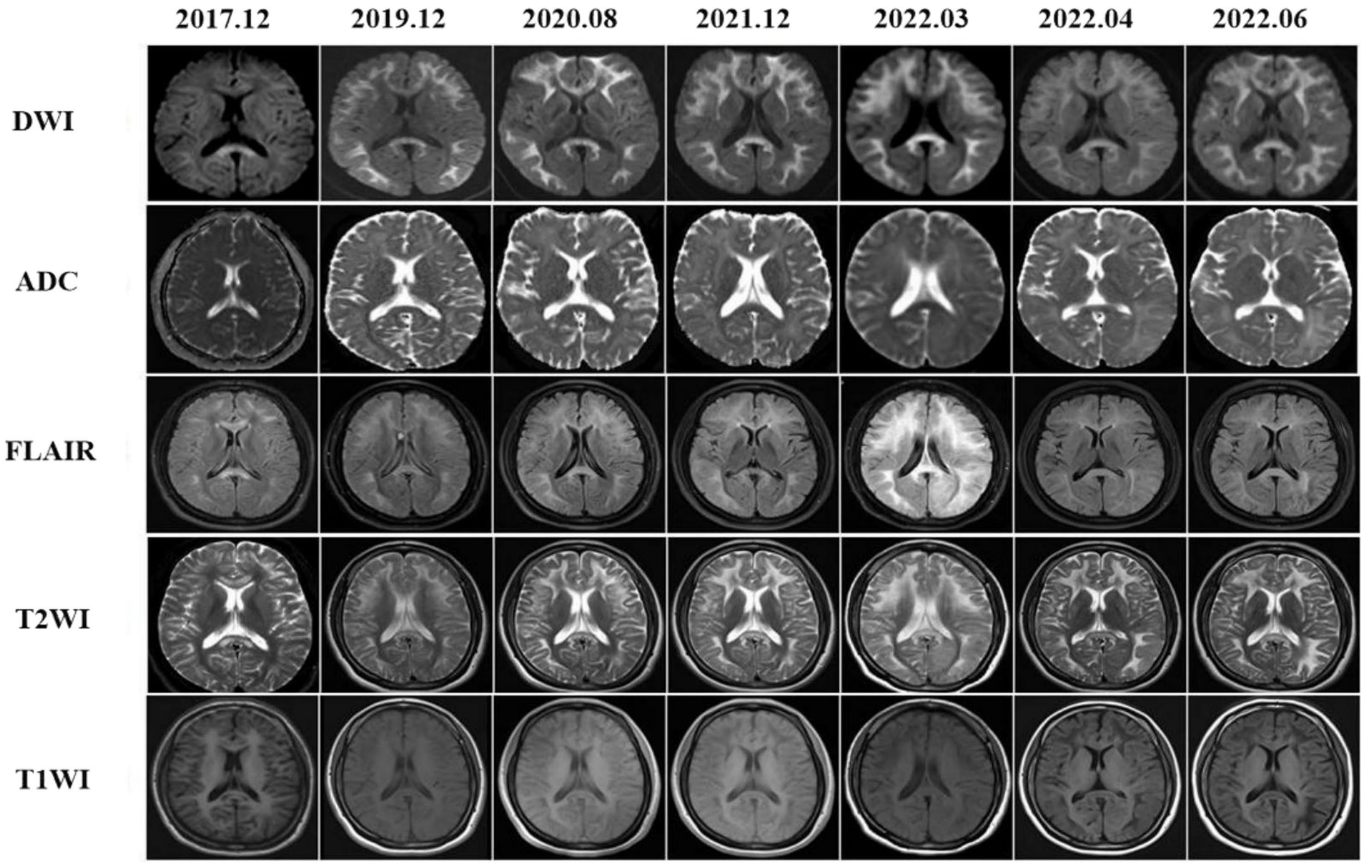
Brain MRI findings showed diffused leucoencephalopathy in T2WI and FLAIR images, there were also hyperintensities in the corpus callosum. On DWIs high intensities were noted within the CMJ, the corpus callosum and external capsules. These abnormal MRI findings were gradually expanded from the CMJ to the deep white matter that were more prominent in DWIs.
